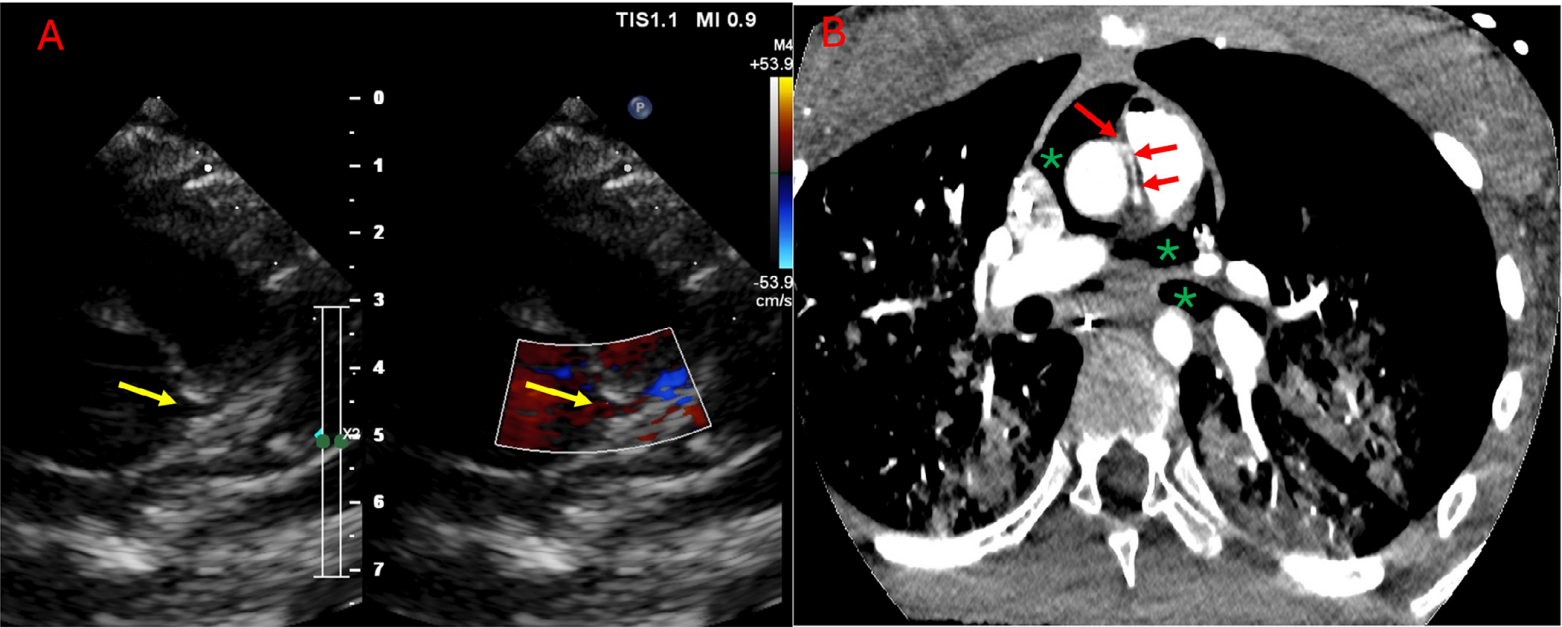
A 13‐years‐old male patient with cardiac arrest during training before a football match. Echo imagines (on the left) acquired when he was in Venoarterial—ExtraCorporeal Membrane Oxygenation seem to capture a normal origin of left main coronary artery in the left Valsalva sinus (yellow arrow). CT (on the right) diagnosed an anomalous origin of left coronary artery from opposite sinus (red arrow) with inter‐arterial course and acute take‐off. * Pneumopericardium (due to resuscitation maneuvers).
